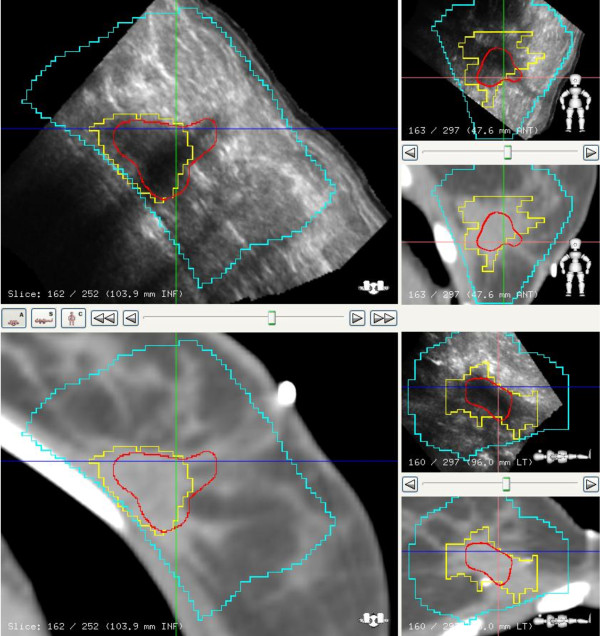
CT image with clips, co-registered with US in axial, coronal and sagittal views. CT cavity (GTV) is in yellow, US seroma (RV) is in red, and blue represents PTV. In this case the US provides additional information for seroma definition.
